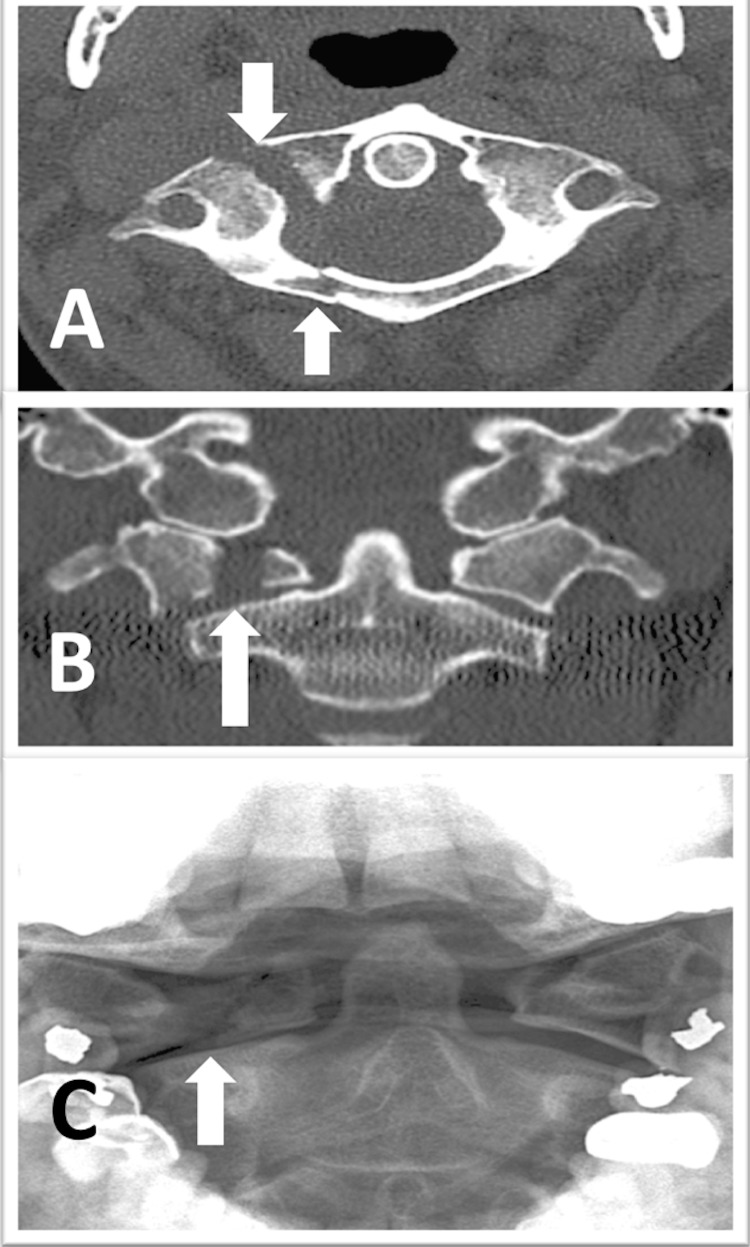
Preoperative images Preoperative images demonstrating the C1 oblique right split fracture (arrows): (A) an axial computed tomography (CT) scan showing an oblique fracture line through the lateral mass and the posterior arch; (B) a coronal CT scan showing a 5 mm overhang of C1 over C2; (C) an open-mouth odontoid x-ray after two weeks of rigid orthosis showing the settling of the C1 right lateral mass
